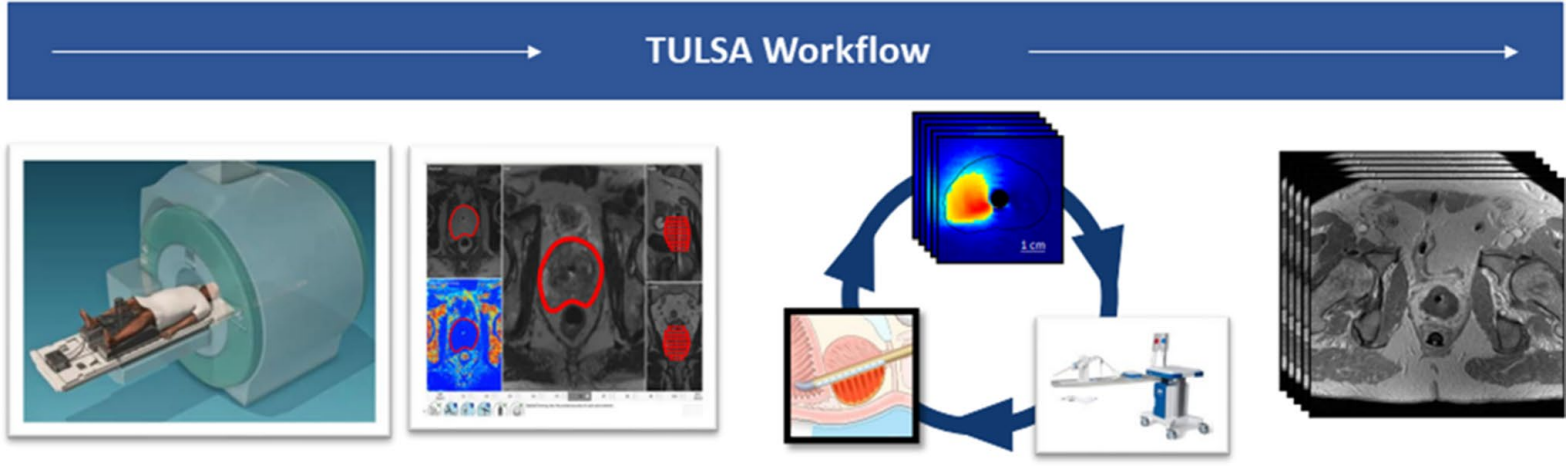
Description of MR-guided transurethral ultrasound ablation (TULSA) device. The transurethral ultrasound catheter ablates prostate tissue “inside out” to induce thermal coagulation. The therapy takes place fully inside the MRI suite, which enables accurate treatment planning and the use of MRI thermometry to monitor the heating. At the very end of treatment, MRI contrast agents are injected into the bloodstream, and the immediate non-perfused volume is compared to the prescribed treatment volume
